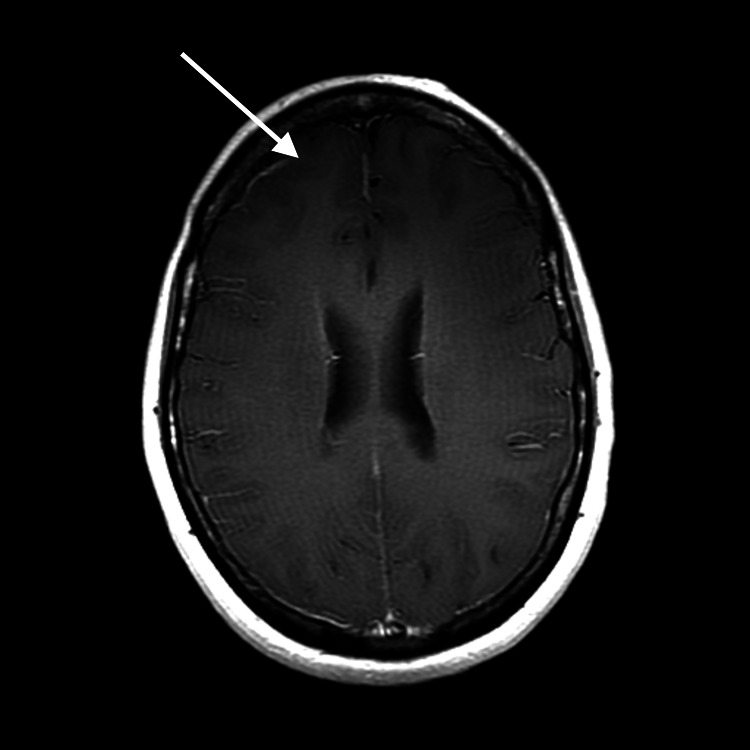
Lack of tumor enhancement (arrow) on post-contrast axial T1 MRI MRI: magnetic resonance imaging
